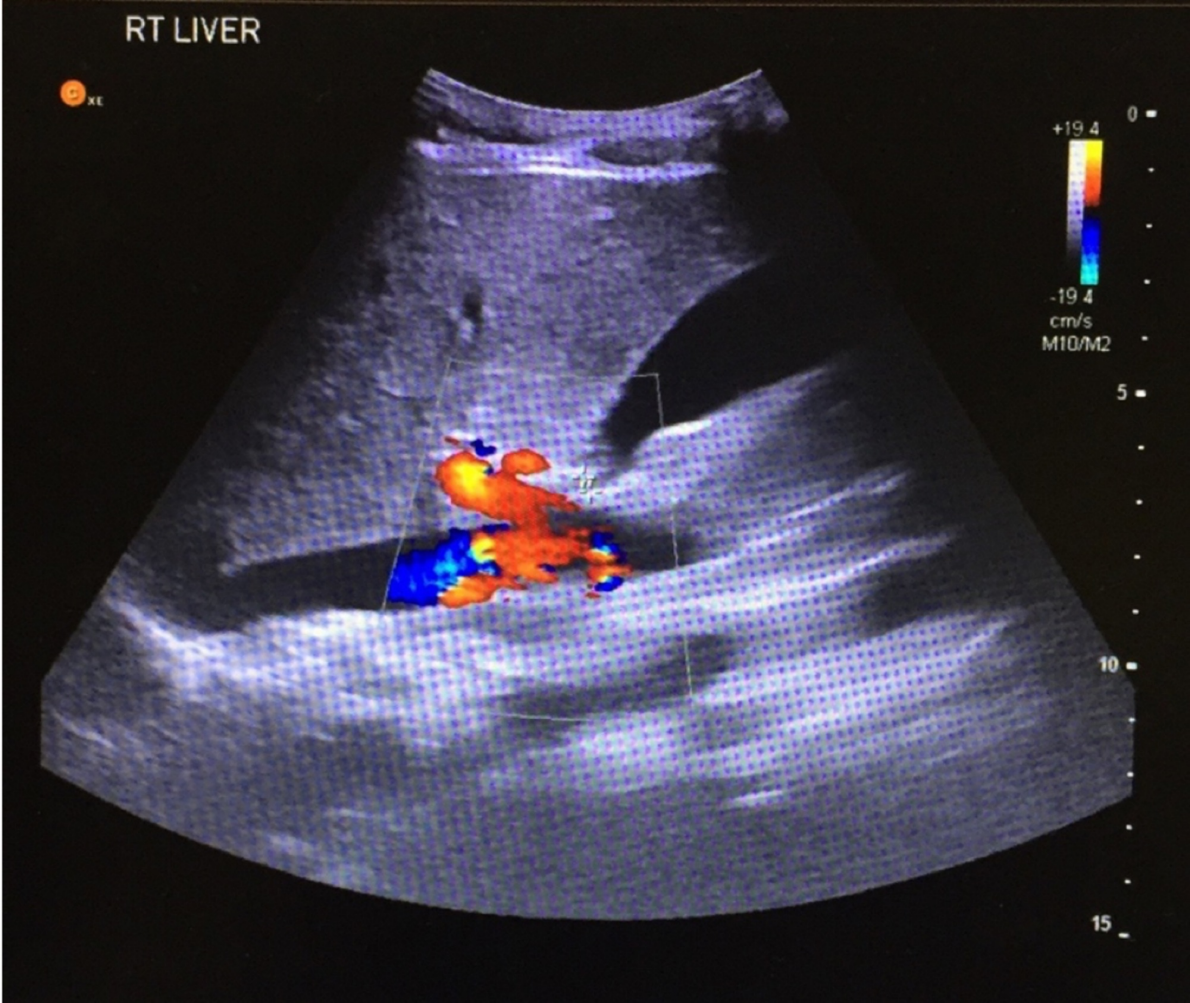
Longitudinal ultrasound visualising appendix at the level of the liver. Uncompressible appendix containing free fluid, measuring 6mm in diameter.
